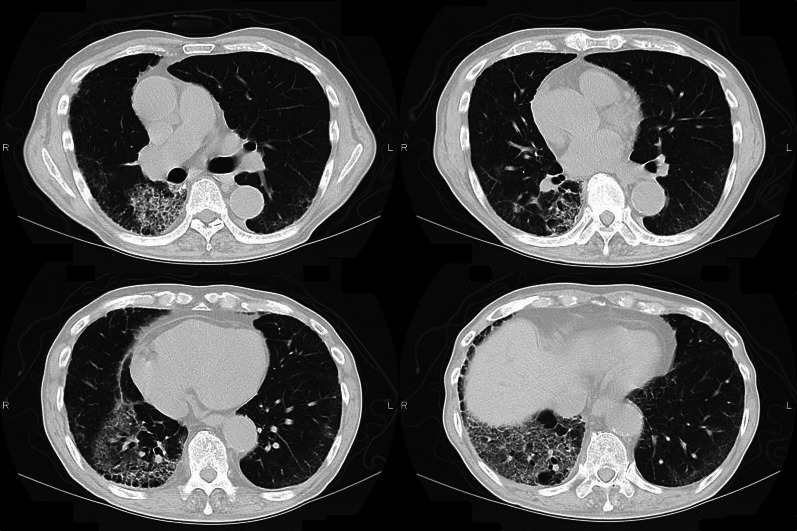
CT; one month before the onset of hemothorax. The invasive mucinous adenocarcinoma occupies a significant portion of the right lower lobe. The mobility of the lung is extremely limited
